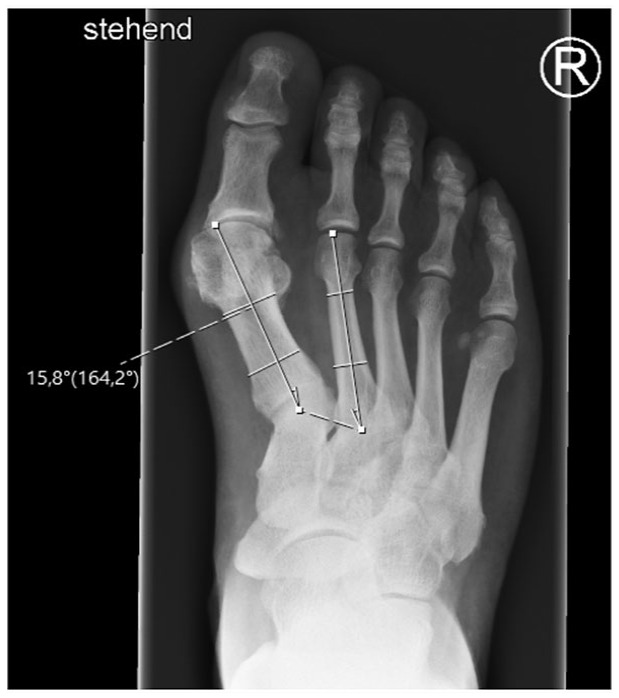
Intermetatarsal angle (IMA): Angle between the anatomical axis of the first and second metatarsal bones on a dorsoplantar radiograph in a standing position with full weight-bearing.
